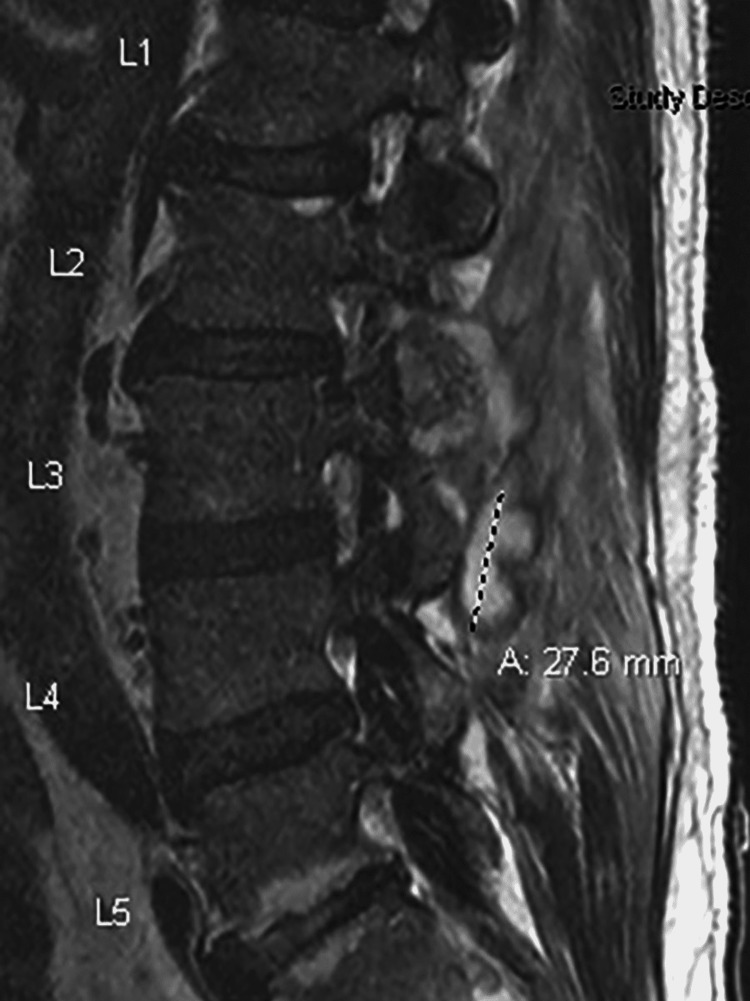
MRI (SAG T2 sequence) showing peripherally enhancing abscess spanning the approximate upper/mid-L3 vertebral body level to the superior L4 vertebral body level, located within the left posterior epidural space of the spinal canal SAG: Sagittal.
